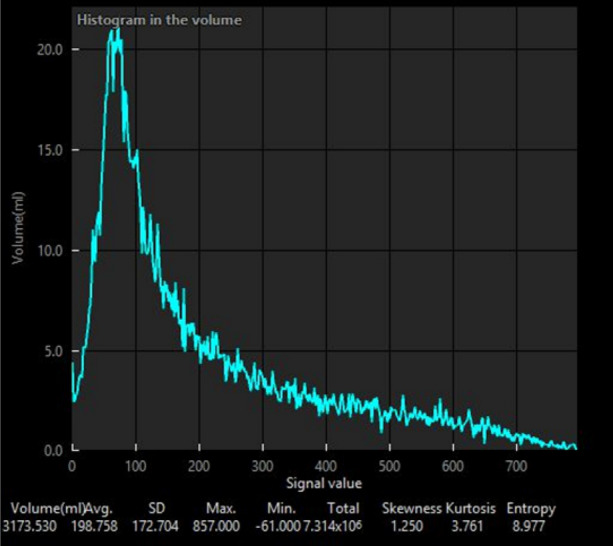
A histogram of radioactivity volume generated from ^99m^Tc-MAA SPECT. ^99m^Tc-MAA SPECT, technetium-99m-macroaggregentated albumin single-photon emission computed tomography
